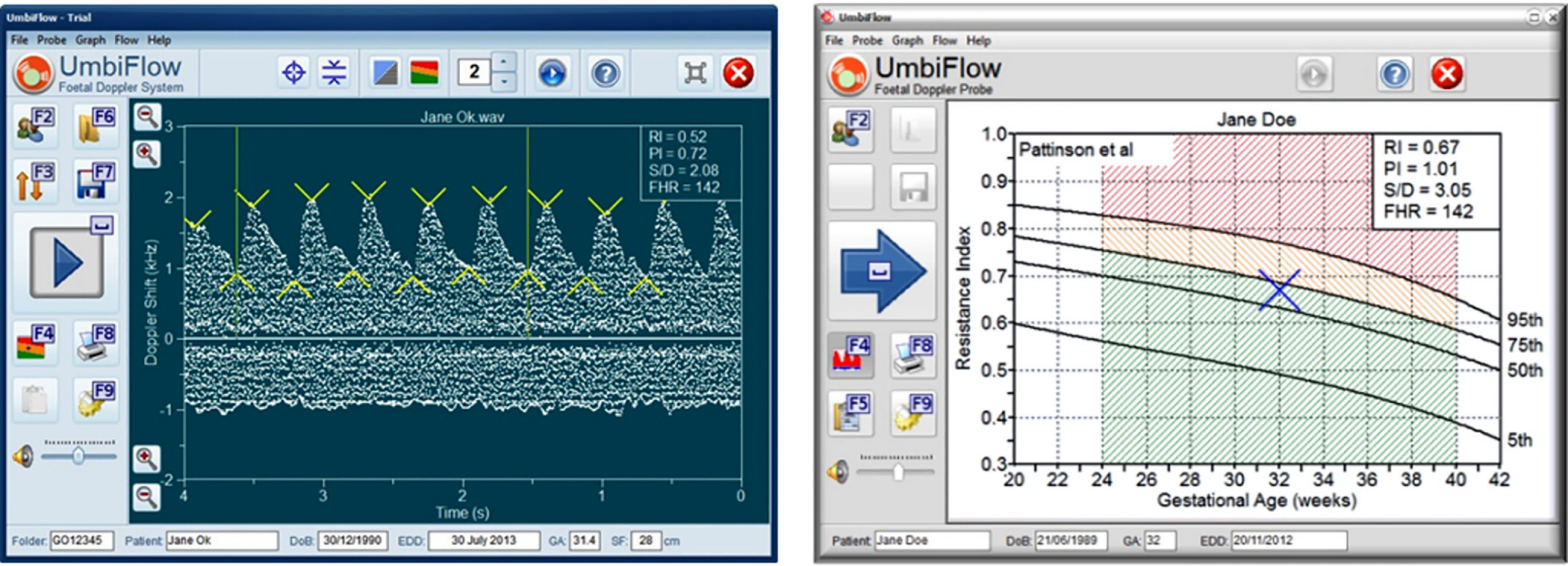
Results from an UmbiFlow™ Doppler measurement plotted against the gestation.
